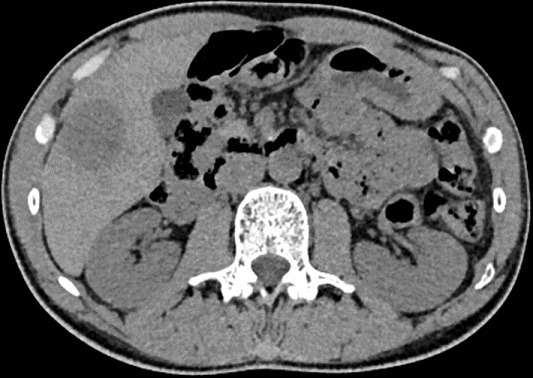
Abdominal CT scan showing a round hypodense lesion localized in the liver segment VI
